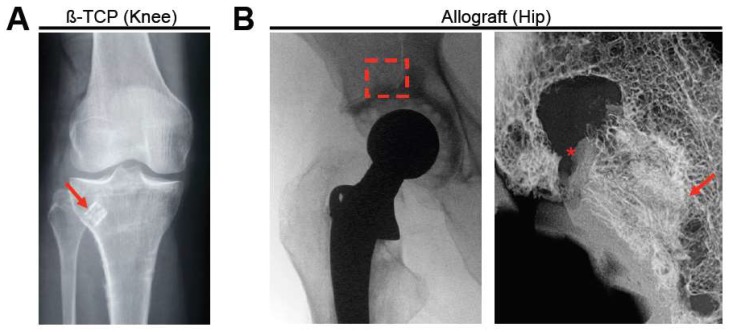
Clinical examples (radiographs) of two different bone substitute types. (A) beta-tricalcium phosphate (β-TCP) block (red arrow) used in a patient with a multi-fragment proximal tibia fracture. Adapted from [1]. (B) Implantation of a structural allograft in revision total hip arthroplasty, left: clinical radiograph (red box indicates the area where the allograft was implanted), right: post-mortem high-resolution contact radiograph. * indicates absent area with no contact, arrow indicates close contact between the graft and the host bone.
